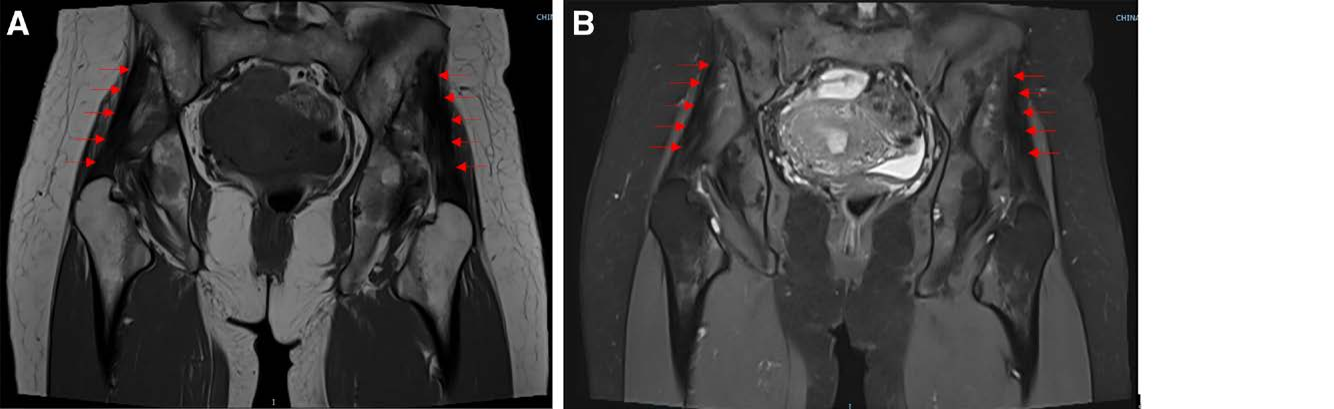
MRI reveals T1-weighted (A) and T2-weighted (B) images, showing significant atrophy of the bilateral gluteus medius, with a low-intensity band within this muscle on both T1- and T2-weighted images (indicated by arrows). These muscular changes are more prominent in the left thigh than in the right. MRI = magnetic resonance imaging.
